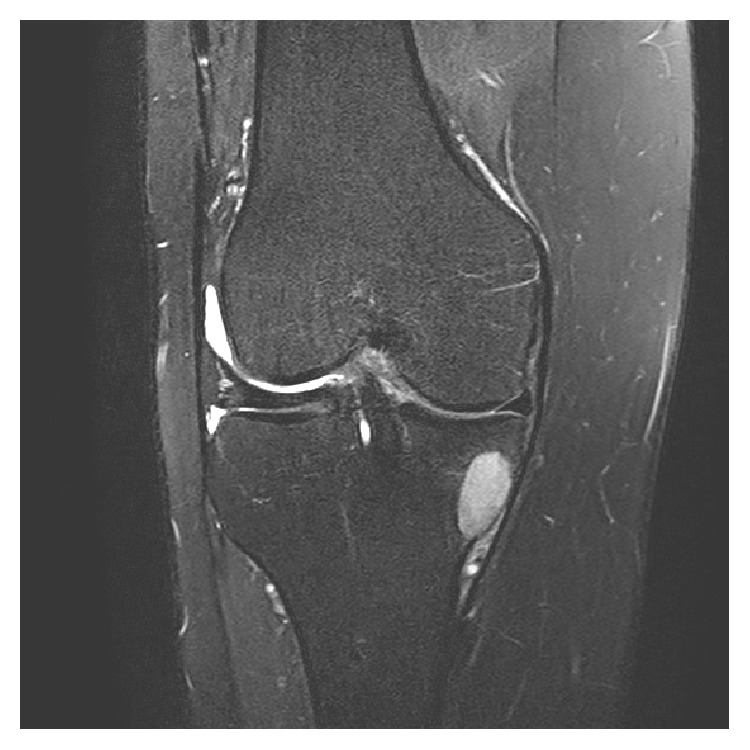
T2 weighted fat suppressed MRI in coronal plane shows well circumscribed hyperintense lesion in the medial tibial plateau without surrounding marrow edema. No communication of the lesion with tibial tunnel was present.
